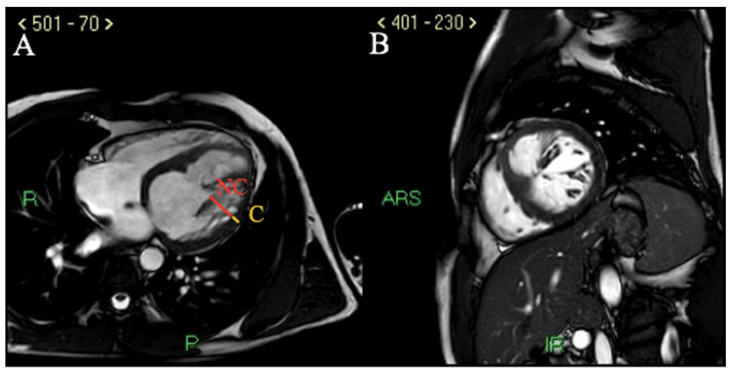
Cardiac MRI. (A) cine-steady state-free precession sequence (SSFP) showing an end diastolic NC/C ratio of 5 in long axis view, diagnostic for pathologic LVNC according to Petersen’s criteria. (B) cine-SSFP sequence showing non-compaction myocardial hypertrabecularization in end diastolic short axis view.
